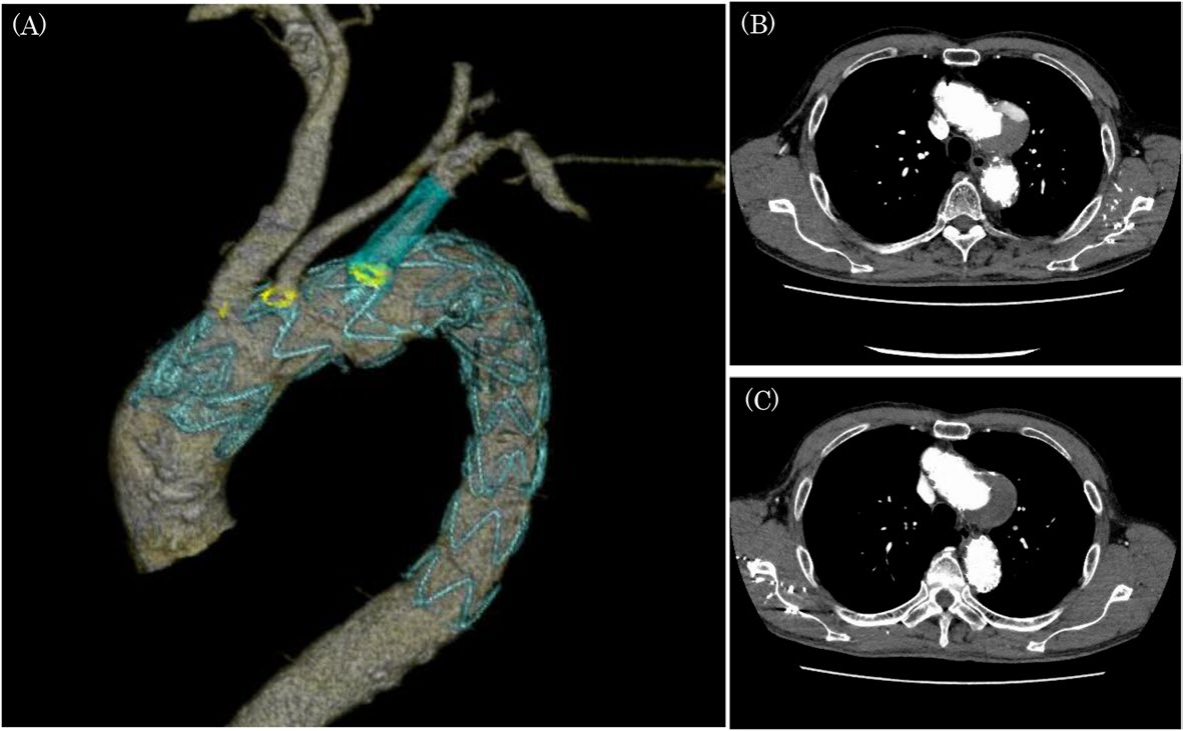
Postoperative computed tomography images. A 3D CT image on the 4th postoperative day. The position of the fenestration completely matched the cervical branch (yellow), and the vertebral artery was preserved. B Contrast CT on postoperative day 4: a type 3a endoleak was suspected at the Viabahn® implantation site. C Contrast CT at 3 months after surgery: the endoleak had disappeared after continuous treatment with 1500 mg of tranexamic acid per day
